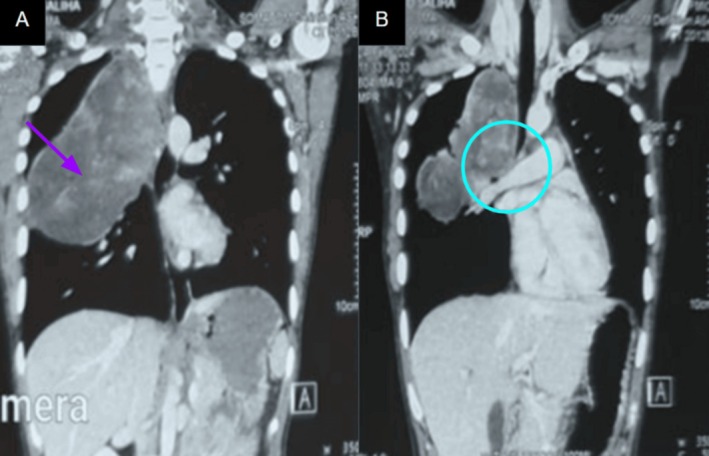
Contrast‐enhanced CT showing the same findings as MRI in Figure 1. (A) A large right upper lobe heterogeneously enhancing mass with internal septations and solid enhancing components predominantly in the periphery of the lesion (purple arrow). (B) It was causing compression upon the right main pulmonary artery and right mainstem bronchus (cyan circle).
